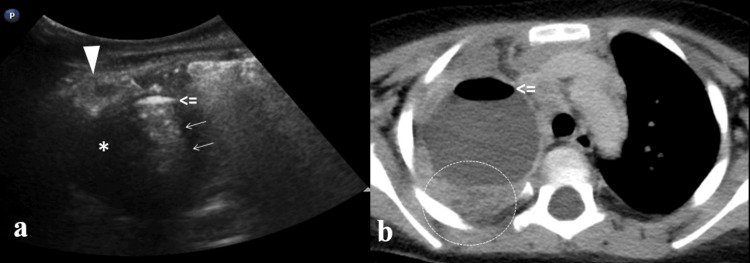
LUS and CT similarities Comparison between lung ultrasound (LUS) (a) and CT (b) examinations. There are a lot of similarities between the images according to depiction of air-fluid level (open arrow) and consolidation (circle). For a more extensive description please see Figure 2.
